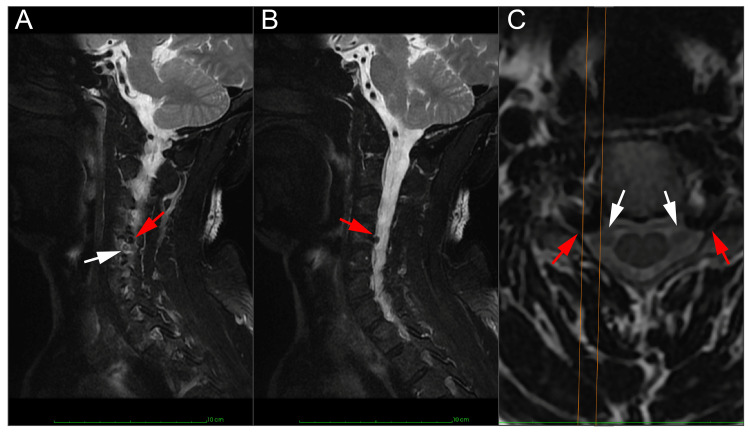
T2-weighted MRI demonstrating bilateral vascular structures at the C4-5 level Bilateral vascular structures (red arrows) at the C4-5 level within the neural foramina, with compression of the right C5 nerve root (white arrows). The bilateral vertebral artery loops are visible as flow voids encroaching into the neural foramina bilaterally.
